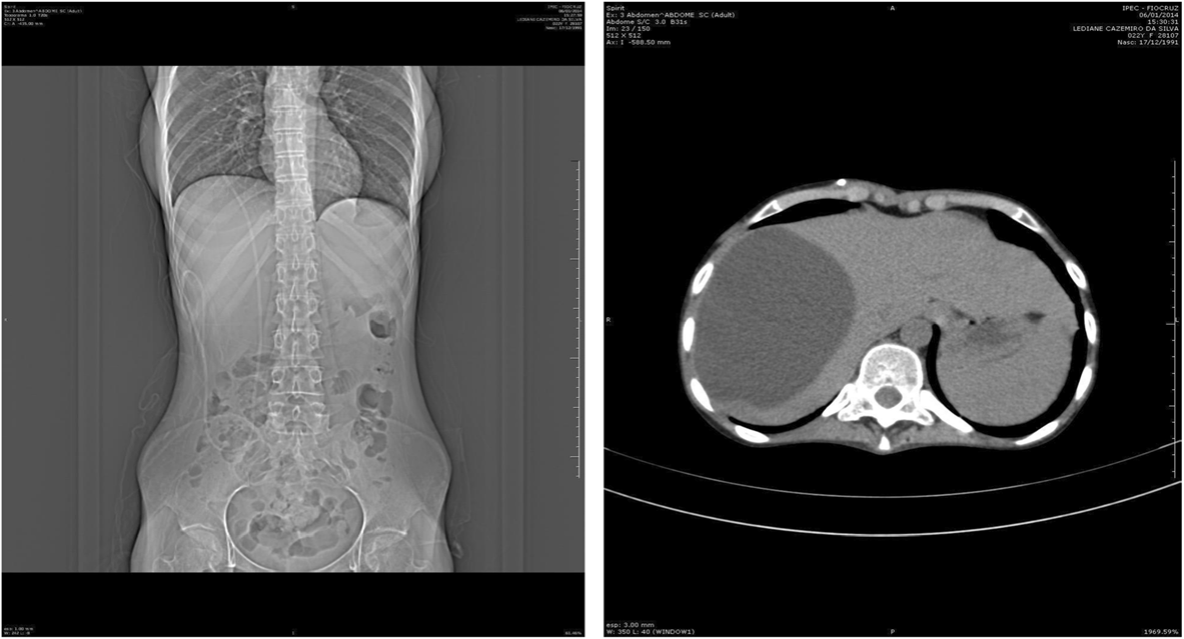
Abdomen CT Scan, January 2014, showing infrahepatic fluid collection (CSF).
